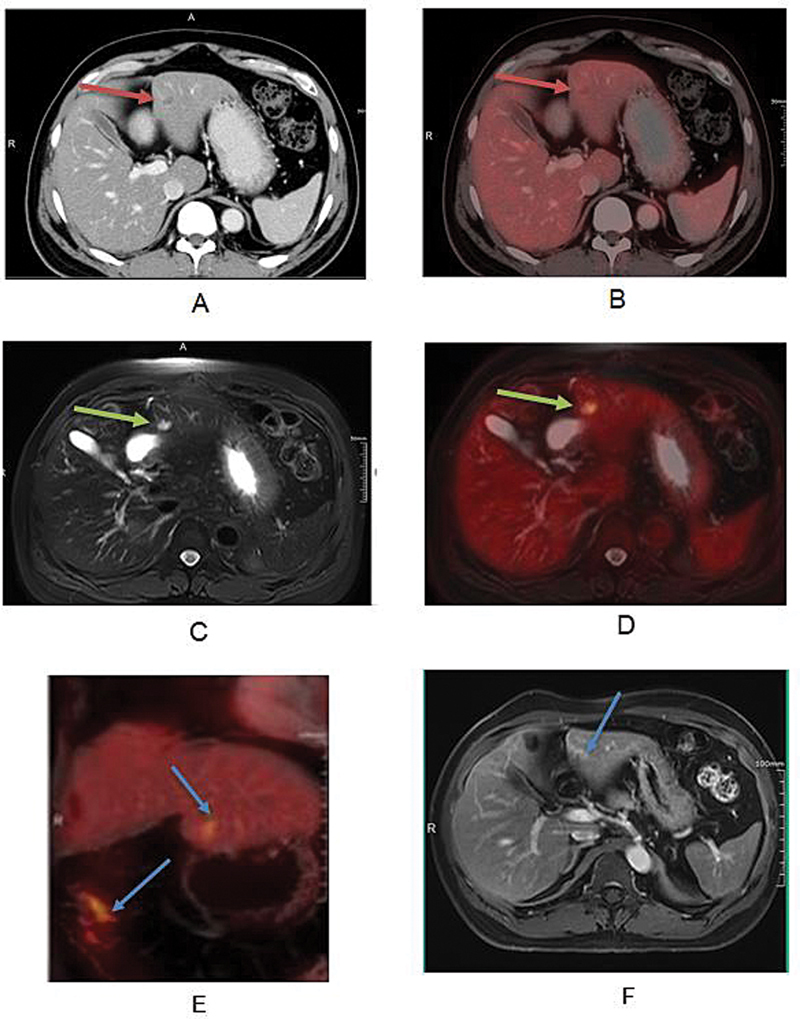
A 48-year-old man with colonic mass and liver lesions: This figure shows the advantage of PET/MRI in revealing additional information about the hepatic mass compared with PET/CT. (
